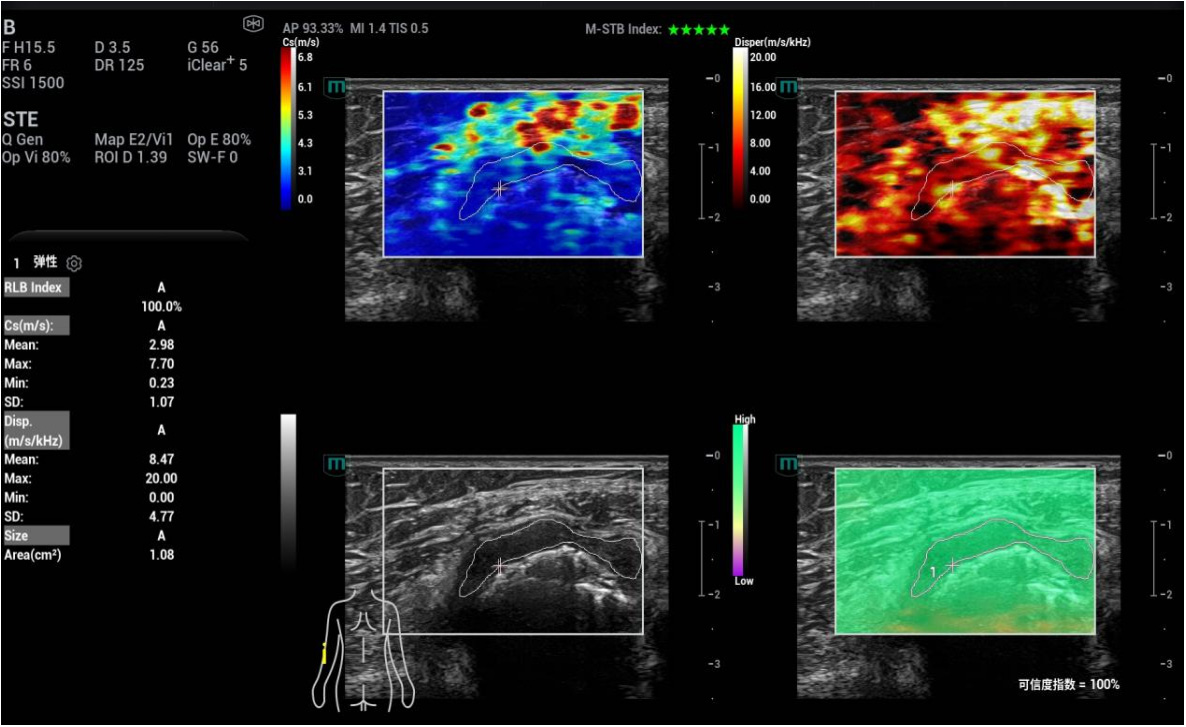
Ultrasound viscoelastic image of the right wrist joint in a patient with rheumatoid arthritis. A 60-year-old male patient. Ultrasound viscoelastic imaging showed that the thickened synovium of the right wrist joint had a Cmean of 2.04 m/s (<cutoff value of 2.36 m/s) and a Vmean of 0.76 Pa⋅s (<cut-off value of 0.81 Pa⋅s).
